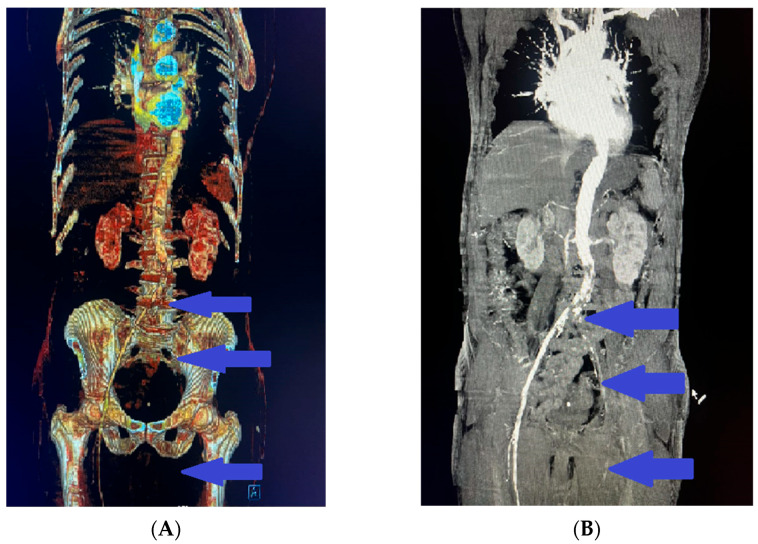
(A,B) Contrast computed tomography (CT) angiography scans revealing complete obliteration and no contrast enhancement in the left iliac artery (blue arrows).
